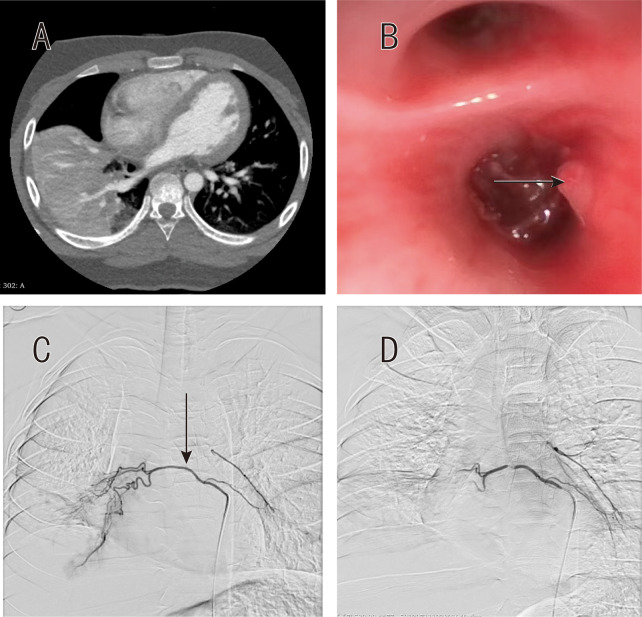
Patient 2 imaging findings. (A) CTA demonstrating right-sided ground-glass opacification without clear vascular etiology. (B) Bronchoscopic view after clot evacuation showing mucosal elevation at right lower lobe bifurcation (arrow). (C) Bronchial arteriography revealing hypertrophied right bronchial artery with early pulmonary venous opacification (arrowhead). (D) Post-embolization image showing successful elimination of arteriovenous shunting.
